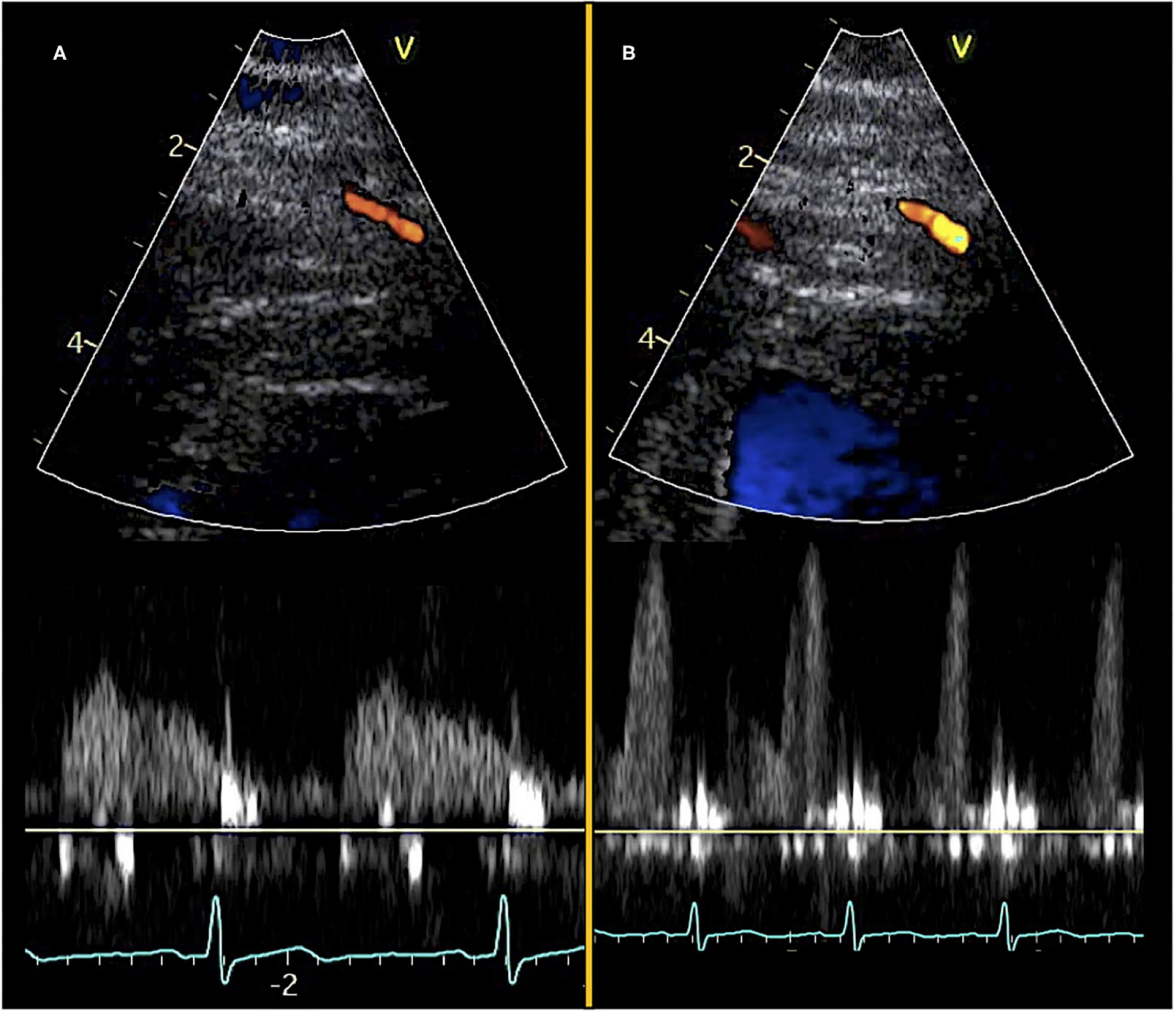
Transthoracic Doppler echocardiography and pulsed-wave Doppler curves. Color Doppler visualization of mid-distal LAD (top) and diastolic pulsed-wave flow velocity curves (bottom). (A) Images obtained at rest and (B) images obtained during adenosine stress.
